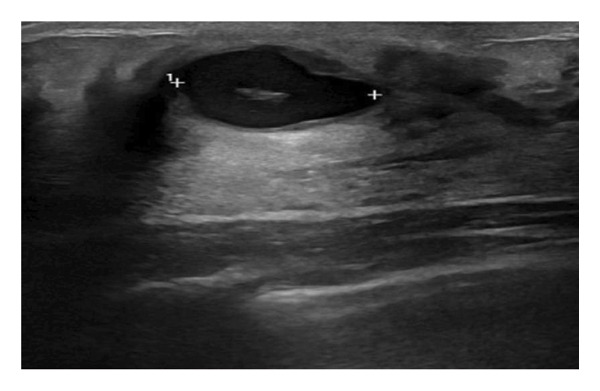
A 12‐year‐old patient presented with right breast pain. The ultrasound shows in the retro areolar area an anechoic nodular formation with posterior wall reinforcement and thickened walls due to abscessation, which resolved after antibiotic therapy.
